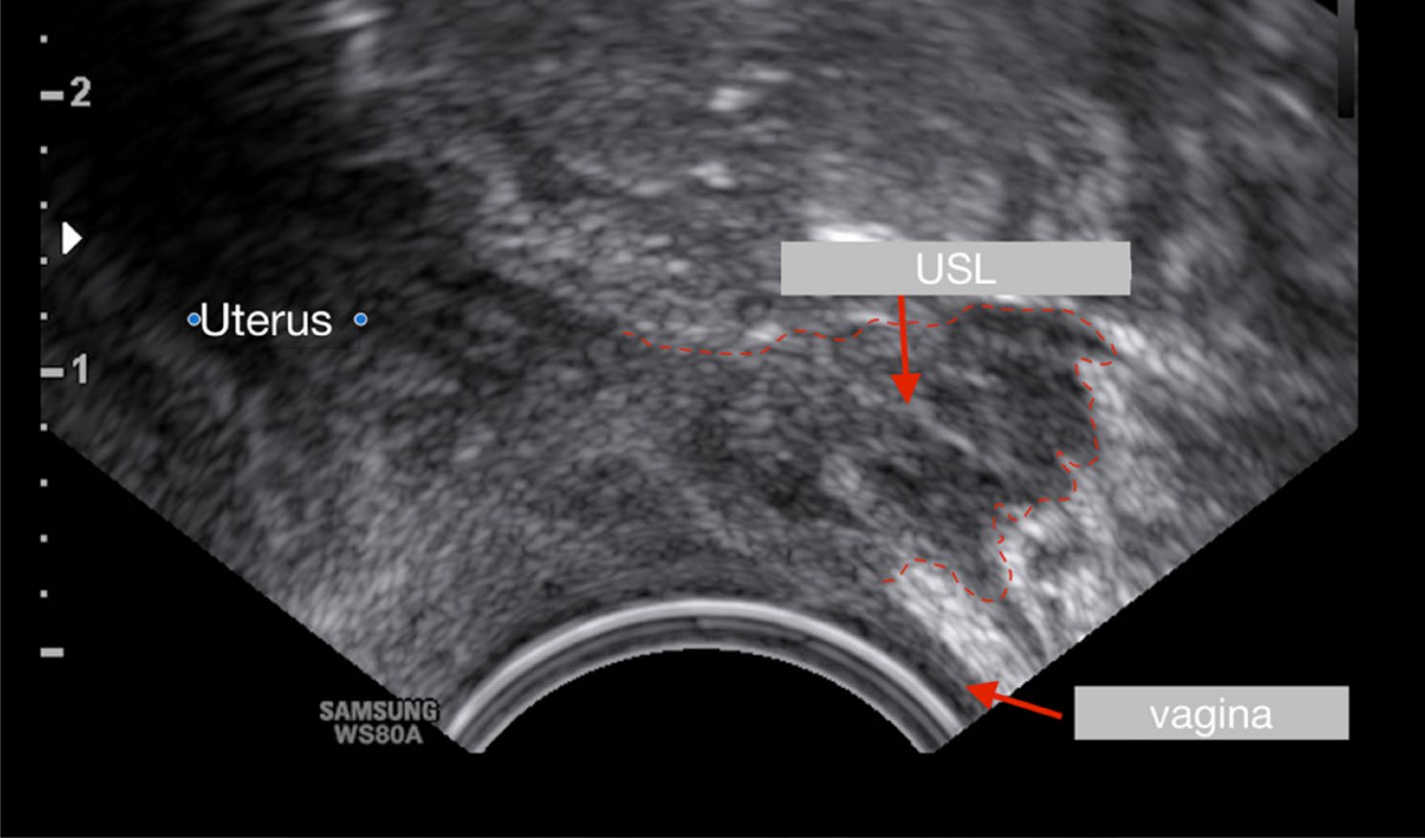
Sonographic image (hypodense areal) of the right uterosacral ligament (USL) (length 1, 2 cm = #Enzian(u)B0/2). The ligament is infiltrated by endometriosis and significantly thickened. In the left part of the image, parts of the cervix uteri are also visible. The vaginal wall is sonographically inconspicuous and has a normal thickness
